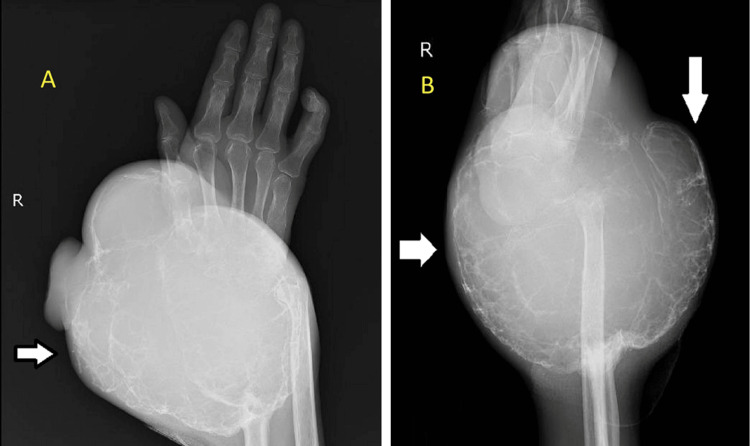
X-ray of the right wrist in anteroposterior (A) and lateral (B) views showing soap bubble appearance
